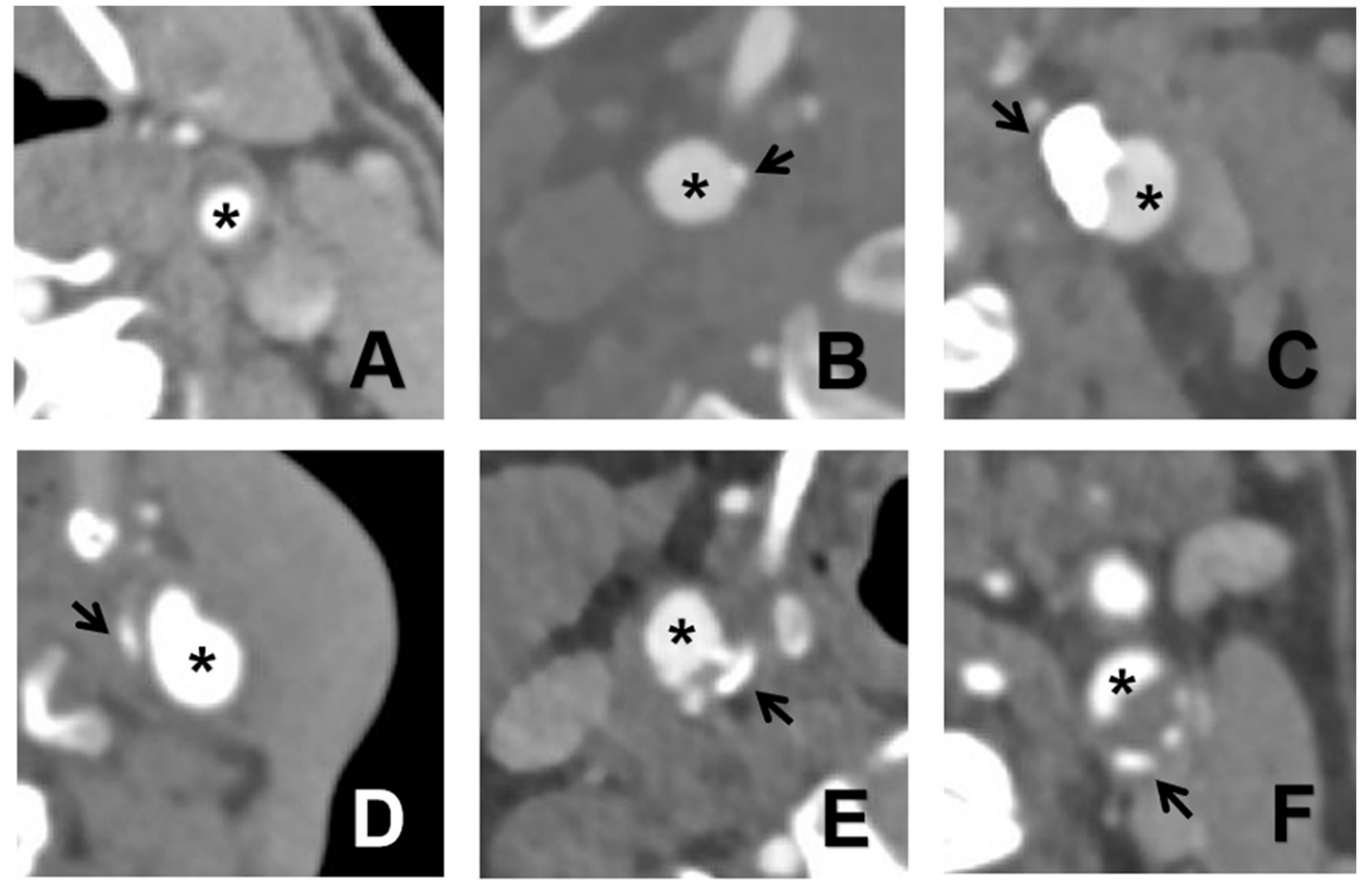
The six types of calcifications on CTA images. (A) no calcification inside the plaque; (B) intima or superficial calcification; (C) deep or large calcification; (D) adventitia calcification having internal soft plaque thickness < 2 mm; (E) mixed type with intima and significant calcification; and (F) positive rim sign. The asterisk indicates a lumen and the *arrow* indicates calcification.
